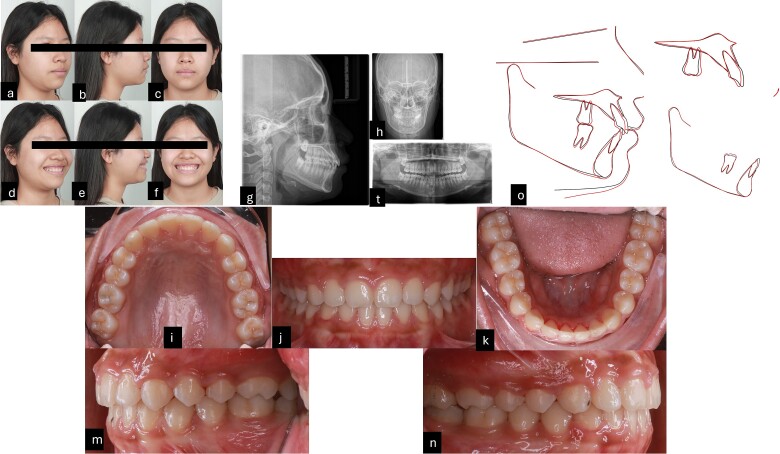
Post-treatment records. (a–f) Extra oral pictures post-treatment; (g–t) post-treatment radiographies; o: superimposition before and after treatment; (i–n) intraoral pictures post-treatment.
